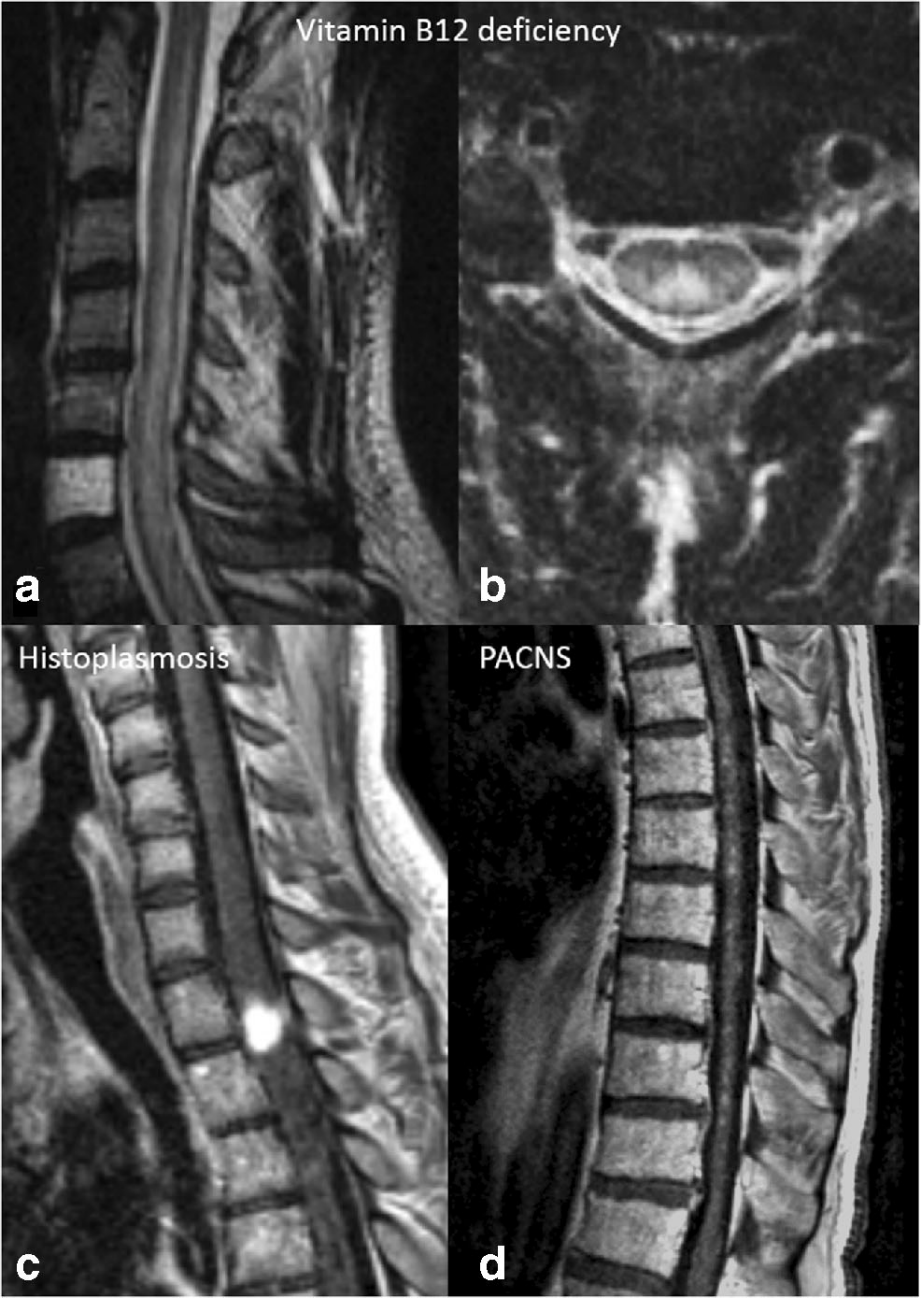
Different patterns of spinal cord involvement. A 39-year-old male with vitamin B_12_ deficiency (see also Fig. 1 for brain images). a Sagittal T2-weighted MRI of the cervical spine showing an extensive multisegmental involvement of the dorsal column of the spinal cord without obvious swelling. b Axial T2-weighted MRI of the cervical spine in the same patient clearly shows the dorsal column involvement of the spinal cord, as a typical finding in vitamin B_12_ deficiency. c Sagittal post-contrast T1-weighted MRI of the cervical and thoracic spine in a 27-year-old male from Central America with lung biopsy–proven histoplasmosis shows a nodular enhancement in the spinal cord at the C7–T1 level. d Sagittal post-contrast T1-weighted MRI of the thoracic spine in a 57-year-old male with brain biopsy–proven PACNS shows a miliary pattern of intramedullary spinal cord enhancement
